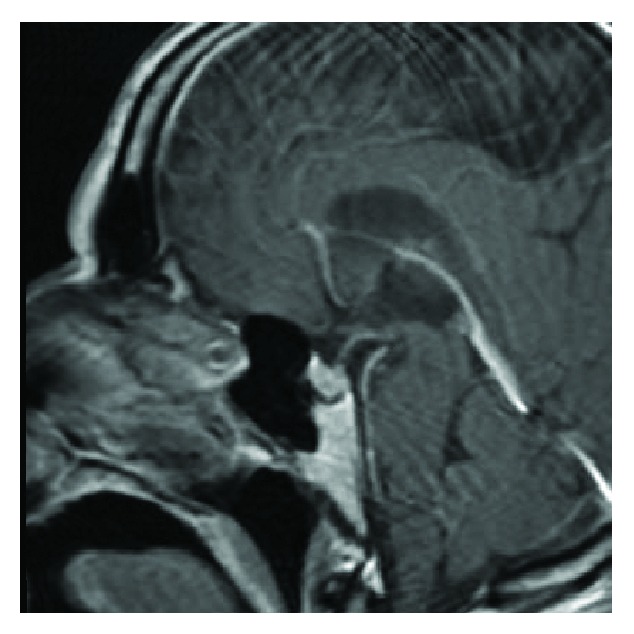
MRI brain, T1 sagittal + gadolinium, revealing complete resolution of all four mass lesions after receiving IV and IT chemotherapy.
